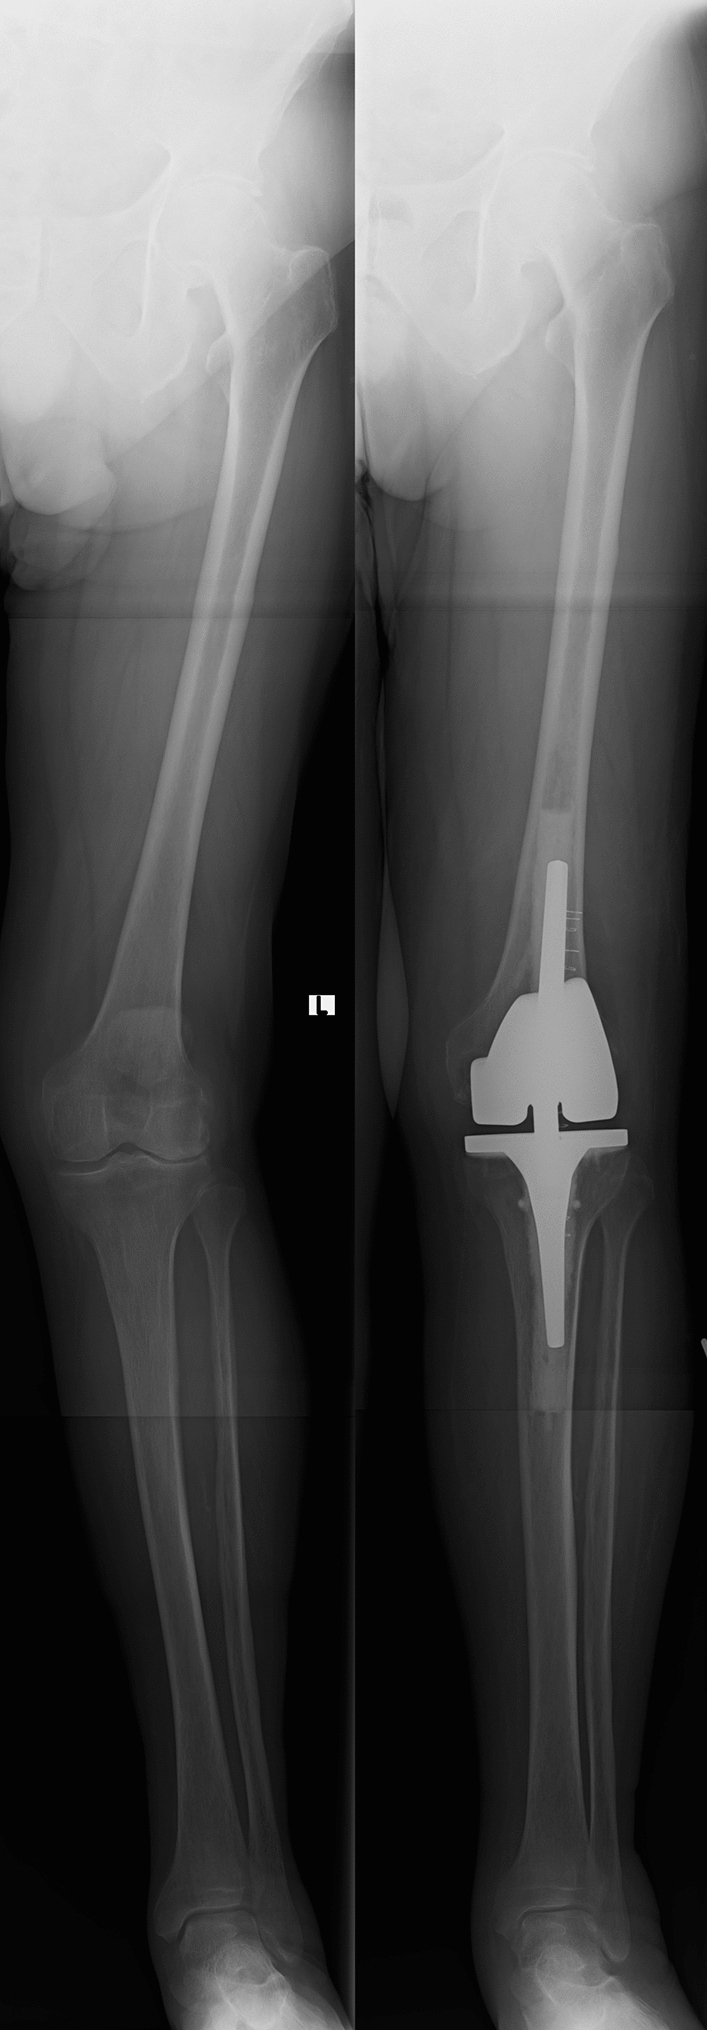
Preoperative (left) and postoperative (right) X-ray of 72-year-old male patient who presented with 16° valgus knee osteoarthritis, which was corrected to a neutral alignment through TKA. Although the G-AJLO was corrected from 10° valgus to 4°, the patient developed ankle pain after the operation (follow-up time 48 months). The LDTA did not change from the preoperative to postoperative period (93°)
